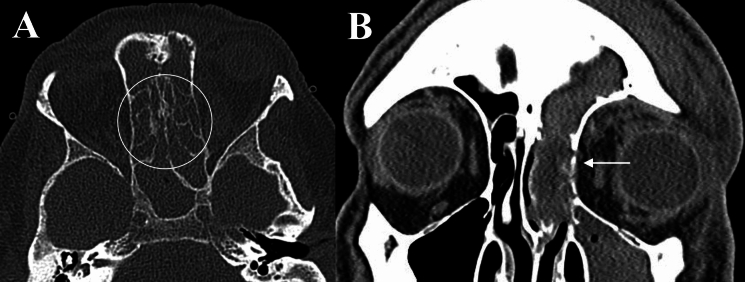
Permeative de-ossification in invasive fungal orbital infections and orbital cellulitis. A Demonstrates permeative de-ossification of the ethmoid sinus in axial CT in a 75-year-old male with invasive mucormycosis of the left orbit (Solid circle). B Depicts de-ossification of the ethmoid sinus and medial orbital wall in a 28-year-old male with left orbital cellulitis (Solid arrow)
